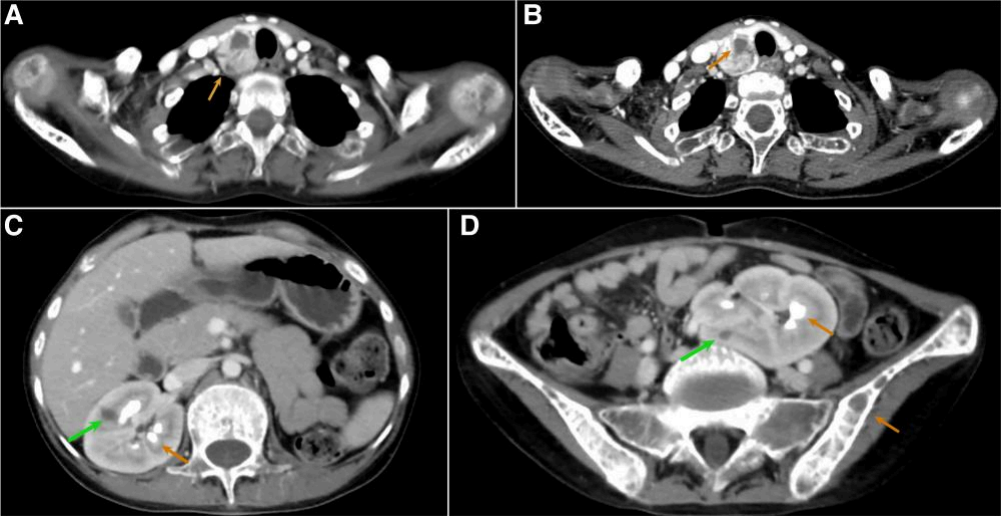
Contrast enhanced CT scan showing (A, B) a large parathyroid lesion arising from the posterior of right thyroid gland, which is intensely enhancing with a cystic component (orange arrow), (C) medullary nephrocalcinosis with a renal cyst (green arrow), (D) ectopic left kidney in pelvis with a renal cyst (green arrow), medullary nephrocalcinosis, and a lytic lesion in iliac bone.
